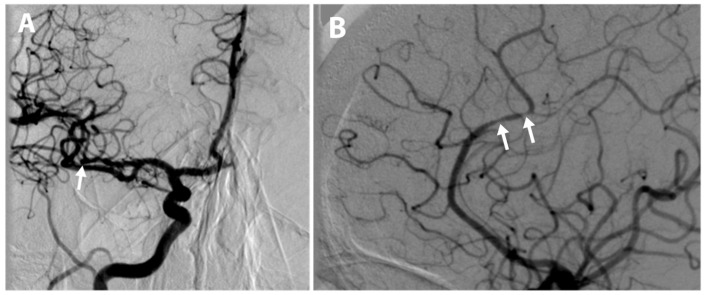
Post-thrombectomy cerebral angiography demonstrating restored blood flow in (A) the right middle cerebral artery, indicated by the white arrow, and (B) the anterior cerebral artery with vasospasm in the A3–A4 segments, indicated by the two white arrows. A follow-up head CT performed immediately after the procedure and 24 h later revealed no signs of acute ischemia or hemorrhage. The post-procedural National Institutes of Health Stroke Scale (NIHSS) score decreased from 10 immediately after the procedure to 5 the following day. On the follow-up day after the intervention, the patient’s neurological symptoms had resolved, with no speech or motor impairments observed, except for mild drowsiness. The patient’s condition upon discharge was stable, and further treatment was continued at the inpatient rehabilitation center.
